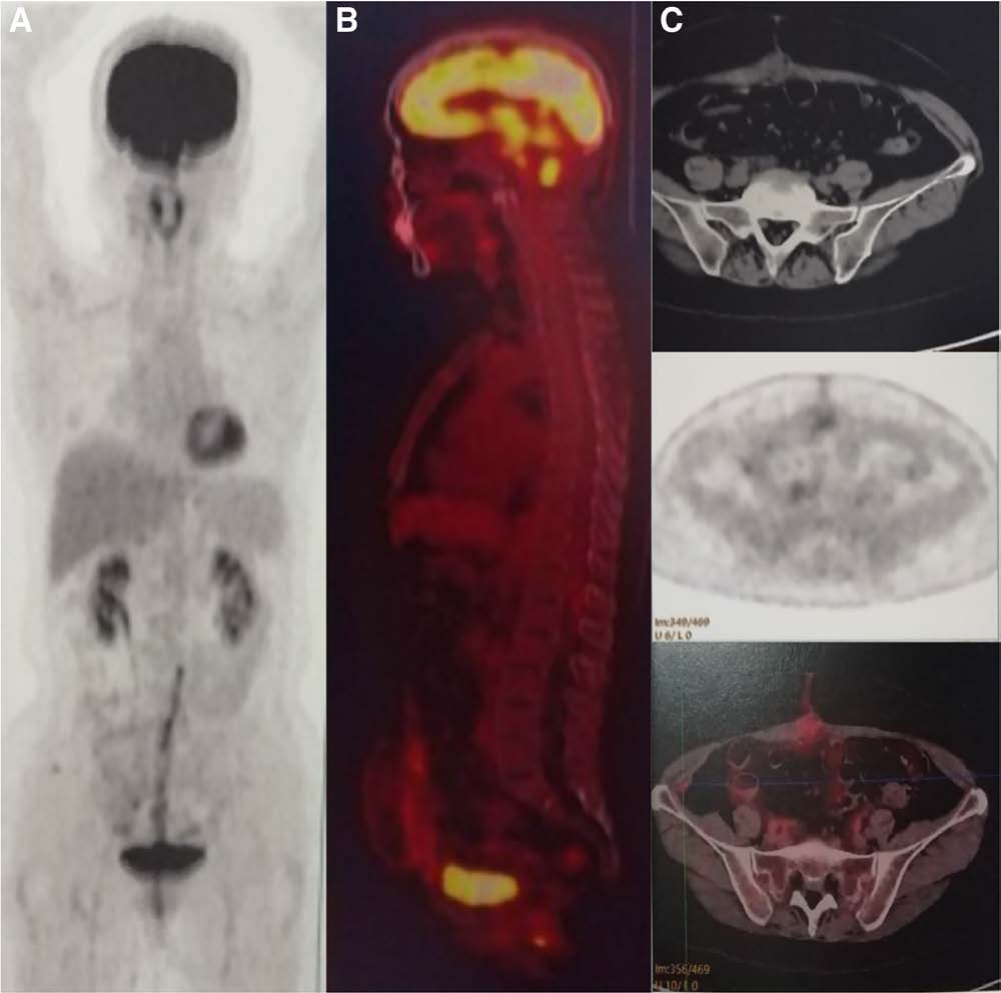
PET-CT showed that there were no abnormal FDG and swollen lymph node in the retroperitoneal and no metastasis lesions on the other organs. (A) The coronal image of PET-CT for the whole body. (B) The sagittal image of PET-CT for the whole body. (C) The cross-sectional image of PET-CT for the abdomen. FDG = fuorodeoxyglucose, PET-CT = positron emission tomography-computed tomography.
